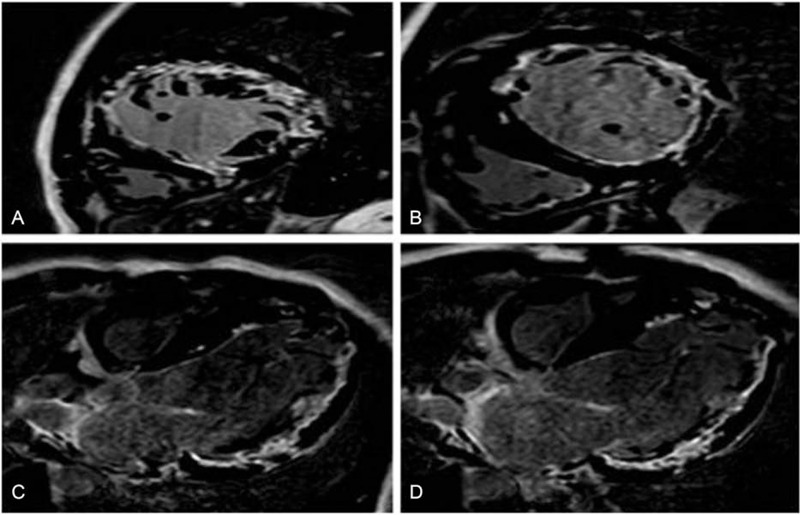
CMRI results. Results of myocardial perfusion, showing a diffuse subendocardial perfusion defect in the left ventricle and delayed enhancement of intracardiac contrast agent emptying. CMRI = cardiovascular magnetic resonance imaging.
